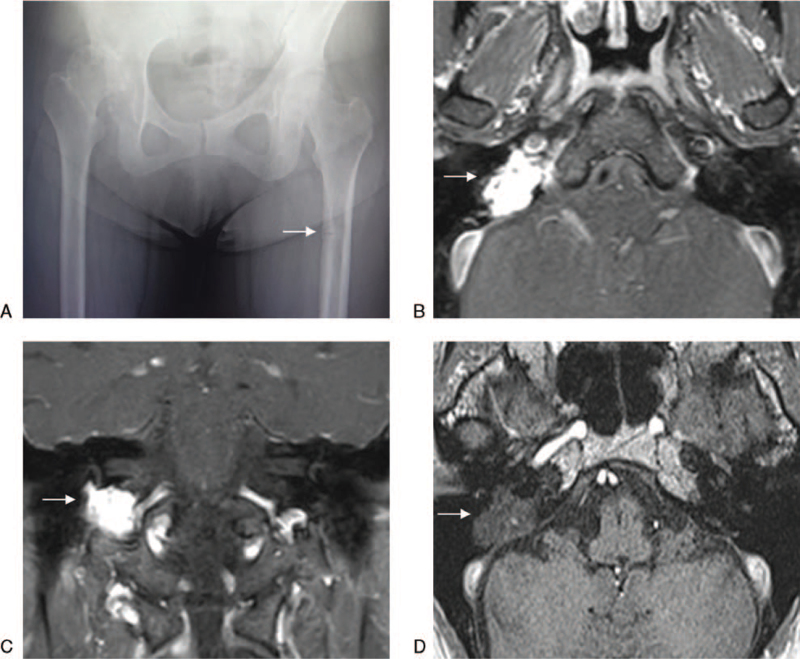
A: Plain radiography of the hip and femur. The arrow represented the mild radiolucent shadow. B: Transverse magnetic resonance imaging (MRI) before radiotherapy, gadolinium-enhanced fat saturation T1-weighted. The arrow represented the tumor and the size was 1.5 × 1.5 × 1.0 cm. C: Coronal MRI before radiotherapy, gadolinium-enhanced fat saturation T1-weighted. The arrow represented the tumor and local invasion. D: Transverse MRI at 5 years after radiotherapy, non-enhanced T1-weighted. The arrow represented the irradiated tumor.
